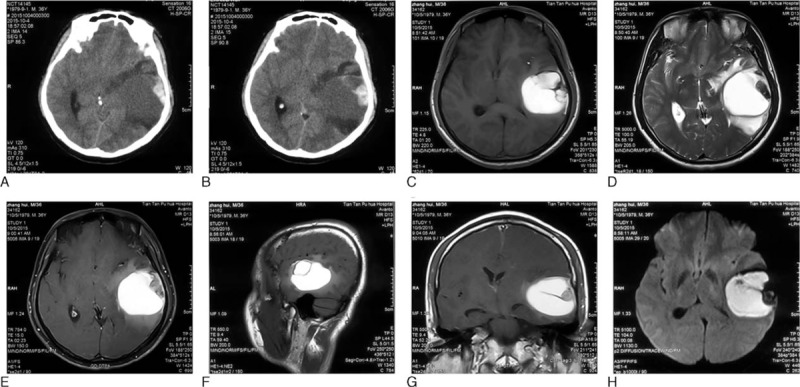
Preoperative brain CT and MRI images. (A and B) Axial view, CT images. (C) Axial view, T1-weighed image (T1WI). (D) Axial view, T2WI. (E) Axial view, contrast-enhanced T1WI. (F) Sagittal view, T1WI. (G) Coronal view, contrast-enhanced T1WI. (H) Axial view, diffusion weighted imaging (DWI). The mass appeared iso-hypodense on brain computed tomography (CT), short signal on T1-weighted magnetic resonance images (T1WI) and long signal on T2WI. It was not easy to make an accurate diagnosis before surgery.
